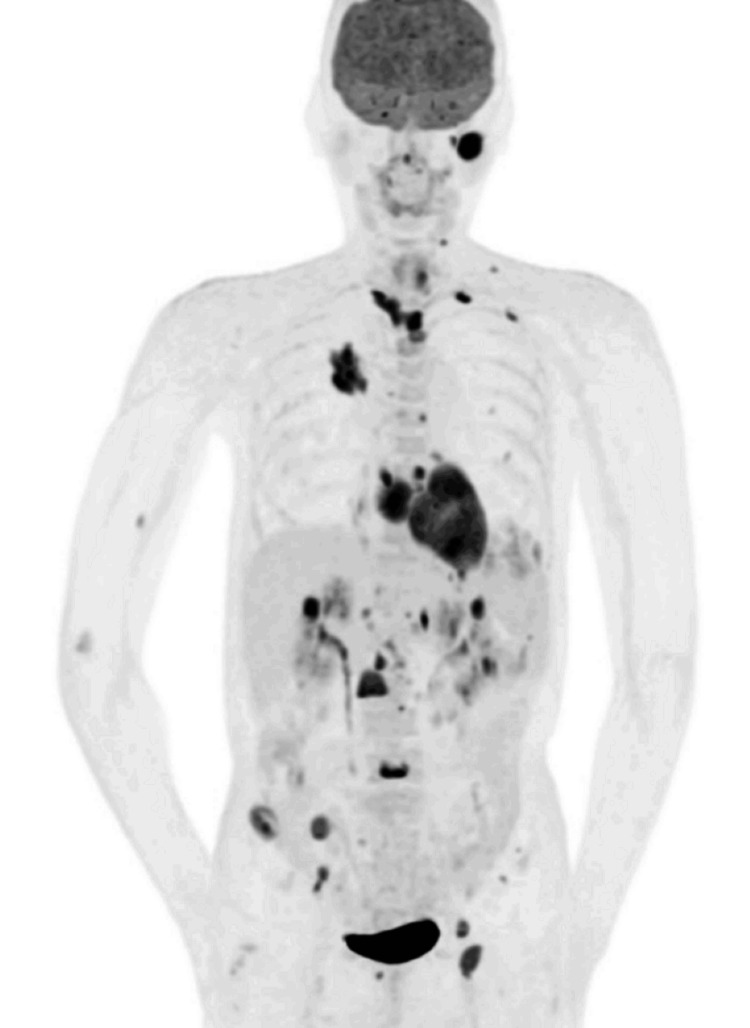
Maximum-intensity projection (MIP) image from ¹⁸F-fluorodeoxyglucose positron emission tomography/computed tomography (¹⁸F-FDG PET/CT) The figure demonstrates multifocal FDG-avid lesions of the brain, left parotid gland, lungs, heart, liver, pancreatic tail, kidneys, left adrenal gland, lymph nodes, and bones.
